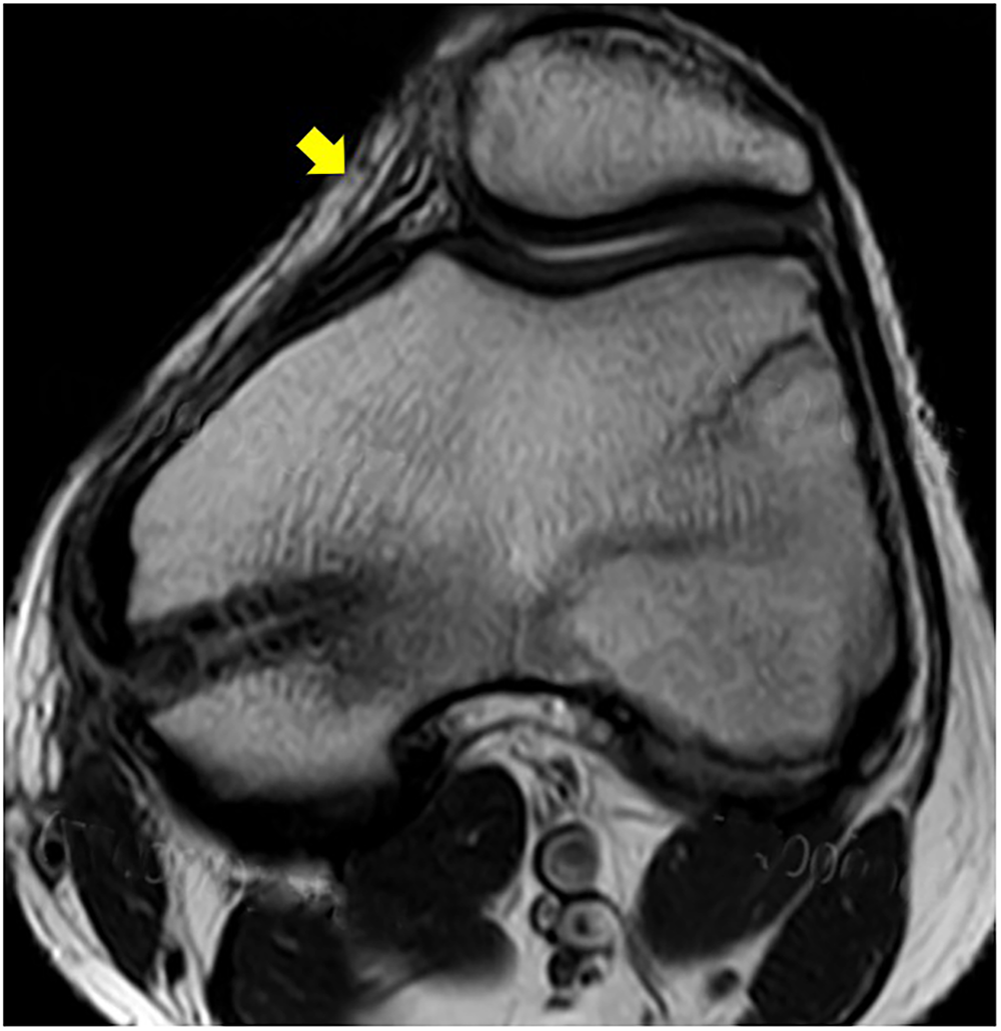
The MRI conducted 35 months after surgery showed a well‐preserved patellofemoral joint and the MPFL (yellow arrow). MPFL, medial patellofemoral ligament; MRI, magnetic resonance imaging.
